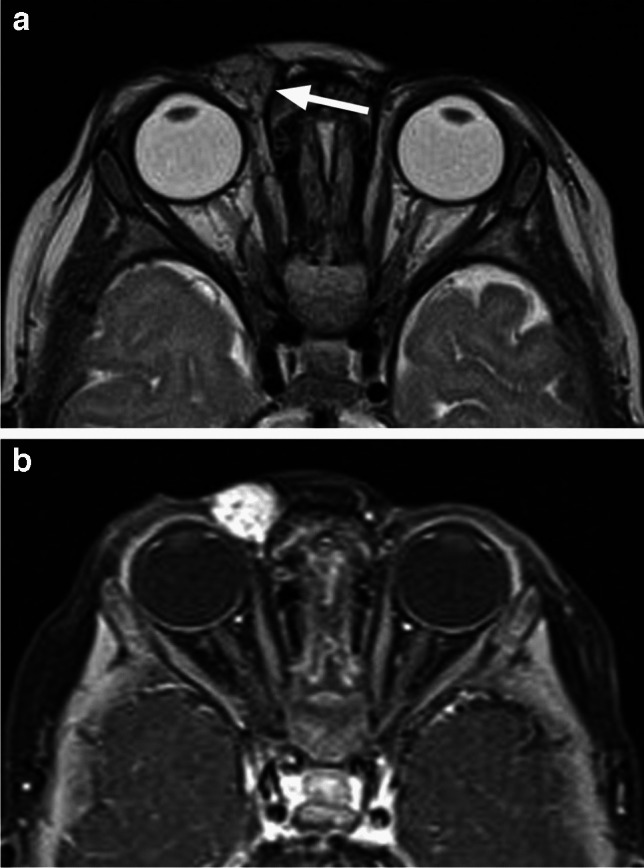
Infantile hemangioma. Axial T2-weighted image through the orbits (a) shows a small preseptal and superomedial extraconal mass (arrow) with internal dark flow voids and fibrous septa. Corresponding postcontrast T1-weighted image with fat saturation (b) demonstrates avid dense enhancement of the well-defined lesion
